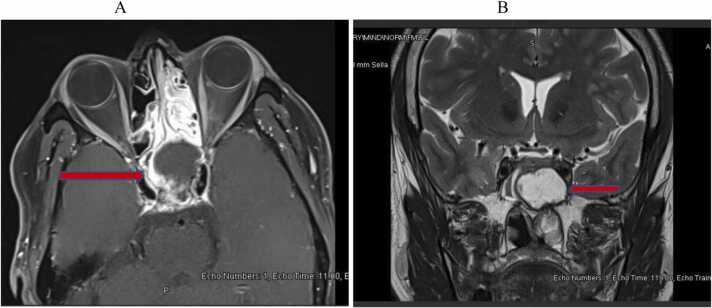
A and B: MRI brain and sinuses revealed a left sphenoid sinus mass (Arrowed) compressing the posterior wall of the clivus, greater wing of the sphenoid, and the optic nerve canal with optic nerve inflammation.
